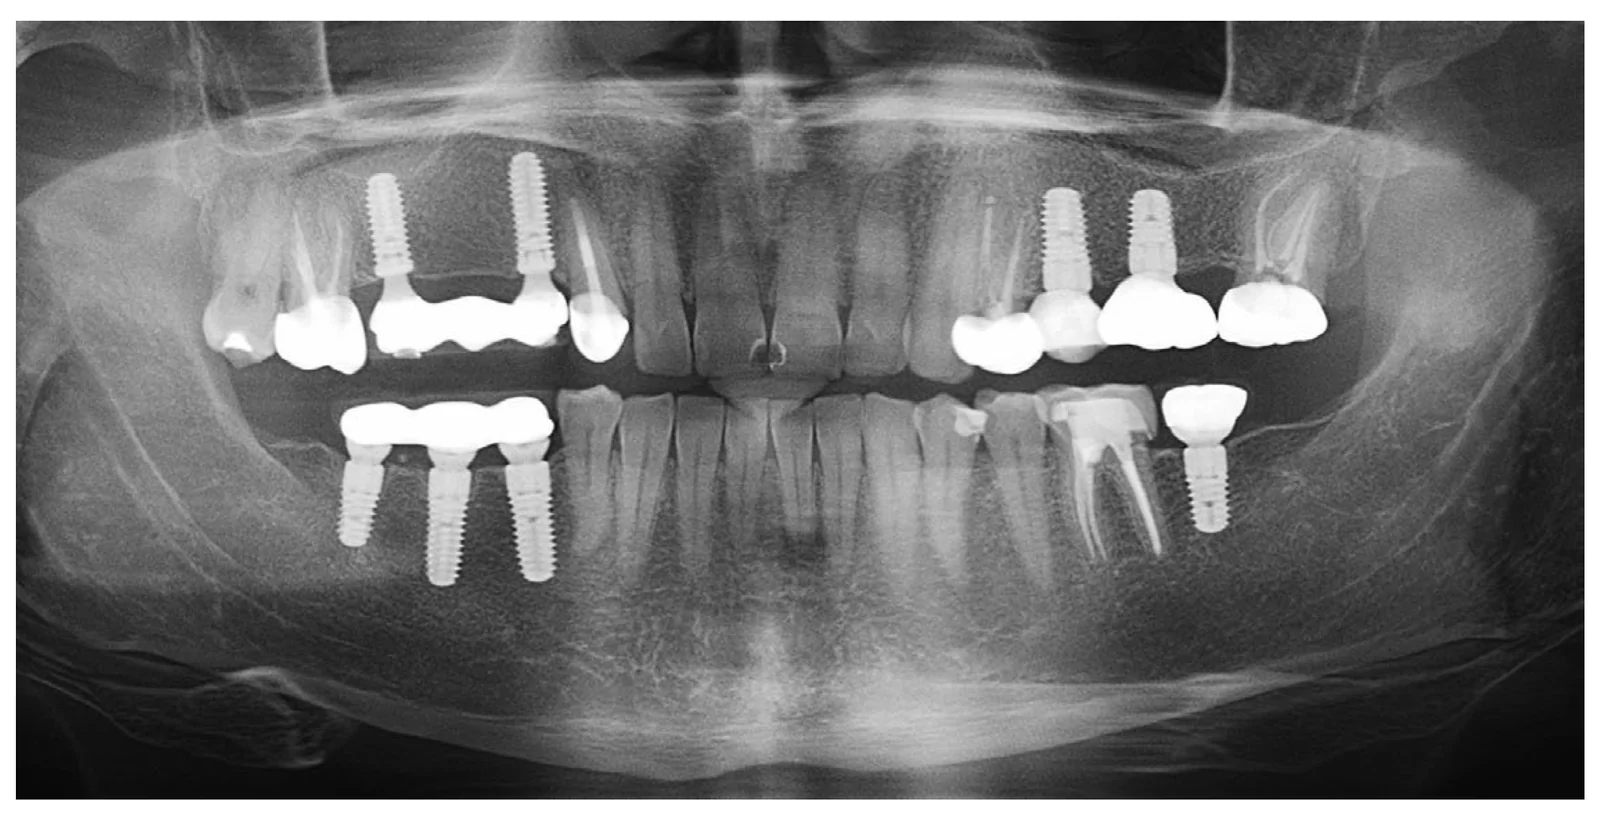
Representative panoramic radiograph acquired using the Planmeca ProMax system.
